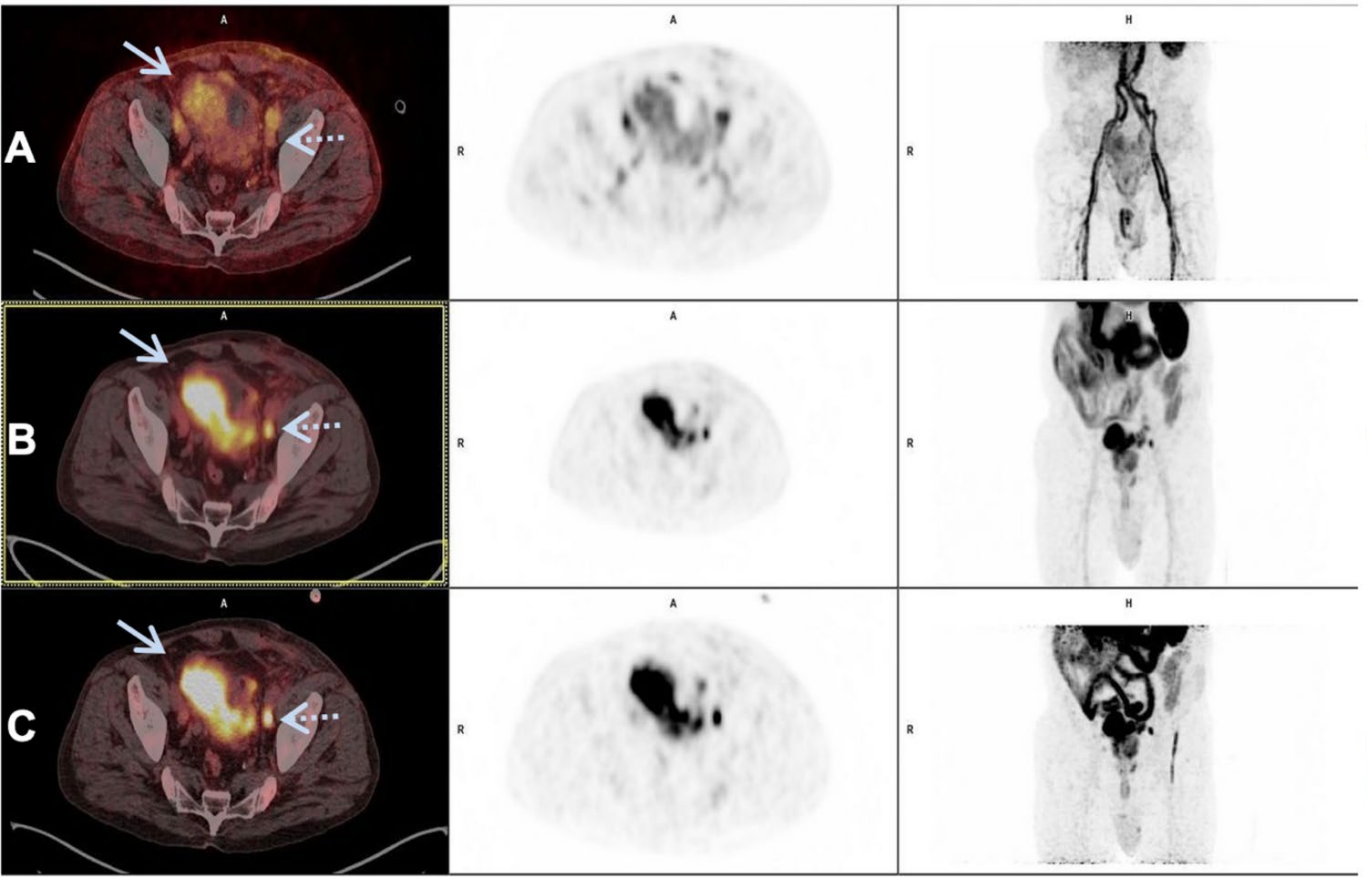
72-year-old, male, T3N1M0, high-grade papillary and non-papillary carcinoma. From left to right: axial fused PET/CT, axial PET, and maximum intensity projection [^18^F]PSMA-1007 images of a patient (patient 2, Table 1) with muscle-invasive bladder urothelial carcinoma and lymph node metastases at (A) 5 min, (B) 90 min, and (C) 2 h after tracer injection. Note the progressive increase in [^18^F]PSMA-1007 uptake in the bladder main lesion (solid arrow) and in a left lymph node metastasis (dotted arrow) from 5 min to 2 h after tracer injection. No significant urinary excretion of [^18^F]PSMA-1007 is seen in this patient.
